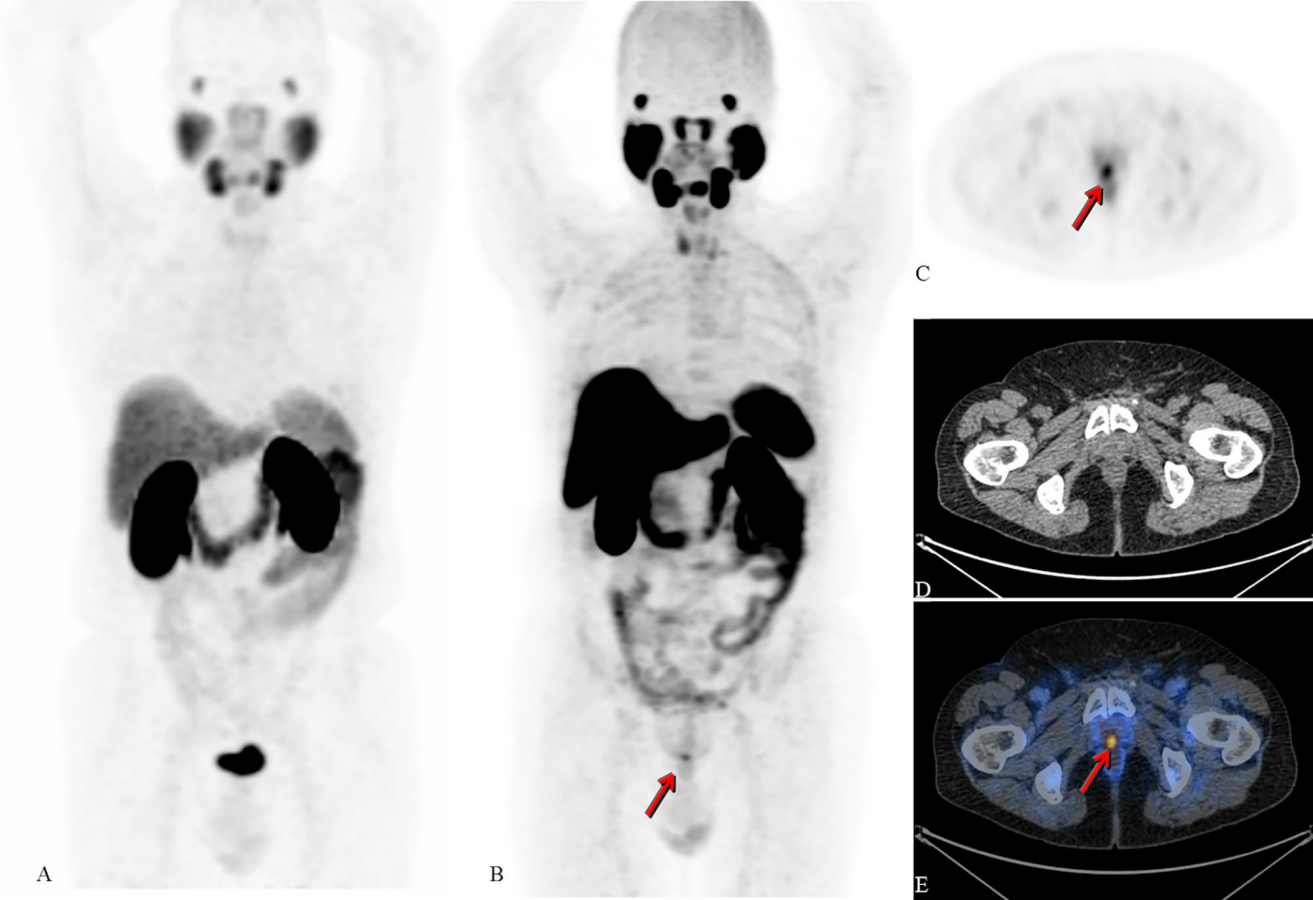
Radical prostatectomy in 2004 due to adenocarcinoma. Salvage radiotherapy due to biochemical recurrence in 2007 with negative restaging on conventional imaging. In 2013, new PSA rise without lesions on restaging with CT, MRI and bone scintigraphy; intermittent androgen-deprivation therapy. Patient referred for 68Ga-PSMA-11 PET/CT in 2016 (not available) and 2017 (maximum intensity projection (MIP); (A) negative for PSMA-expressing lesions (PSA levels at 0.8 and 1.0ng/mL, respectively). Due to further increase of PSA (1.1ng/mL) an 18F-PSMA-1007 PET/CT examination took place in 2018. Images B - E show 18F-PSMA -1007 PET/CT of the same patient (B): MIP, (C): axial cross section PET, (D): axial cross section CT, (E): fused axial PET/CT image). Arrows show unequivocal PSMA-expression corresponding to local recurrence; the quality of the image is not biased by PSMA-detection in the ureters or inside the bladder (in comparison to A) [republished with permission of the Hellenic Journal of Nuclear Medicine (7)].
